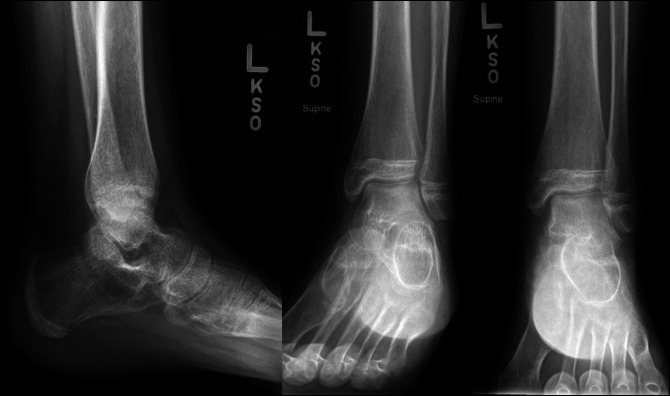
AP, mortise, and lateral view ankle radiographs 12 weeks after the injury demonstrating complete healing of fractures.
